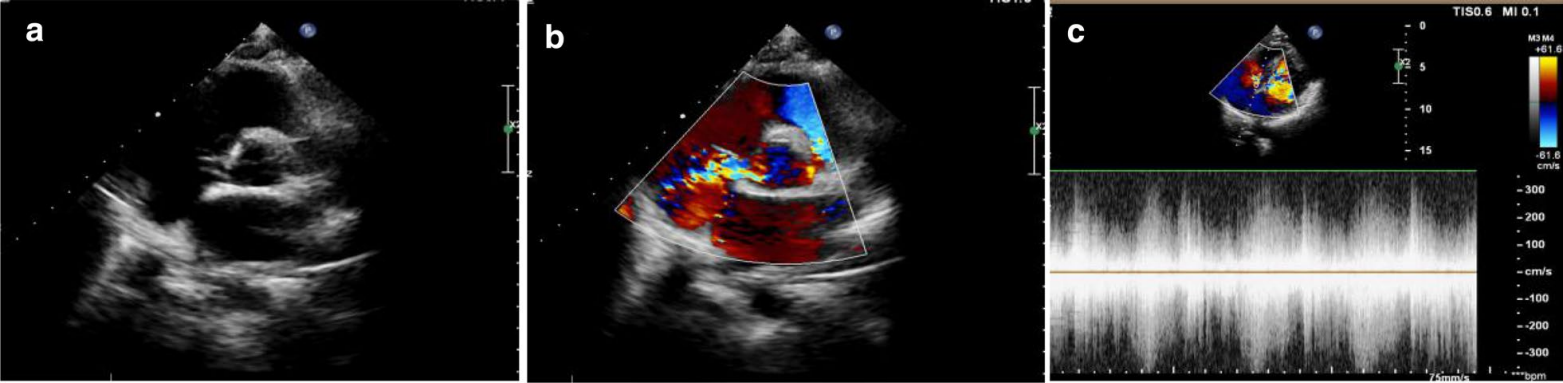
Transthoracic echocardiograms in one patient. a Two-dimensional and b colour-flow Doppler modes show the rupture of the noncoronary sinus into the right atrium. c Continuous-wave spectral Doppler mode shows continuous flow through the ruptured aneurysm
